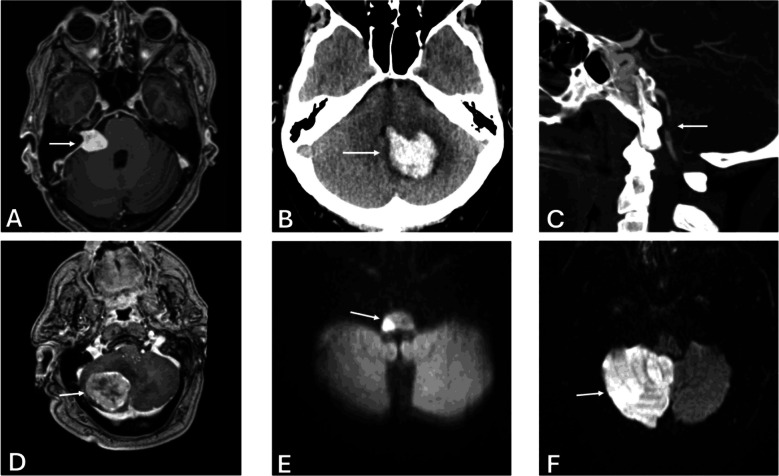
Neuroimaging examples of actionable pathologies detected in patients presenting with vertigo. (A) Right-sided vestibular schwannoma (axial contrast-enhanced T1-weighted MRI); (B) Left cerebellar intraparenchymal hemorrhage causing fourth ventricular compression (non-contrast CT); (C) Occlusive thrombus in left vertebral artery V4 segment (sagittal CTA maximal intensity projection); (D) Right cerebellar metastasis with mass effect (axial contrast-enhanced T1-weighted MRI); (E) Right lateral medullary infarct (axial DWI MRI); (F) Extensive right cerebellar infarct (axial DWI MRI)
